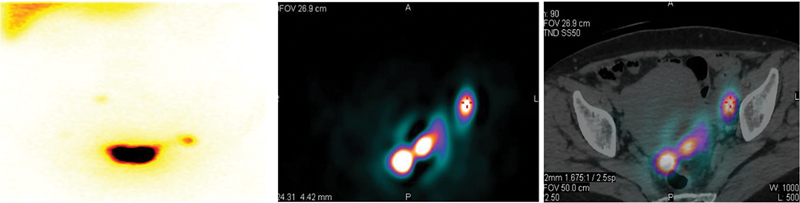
Localization of sentinel lymph nodes on single photon emission tomography/computed tomography (SPECT/CT) images in endometrial cancer patients.
